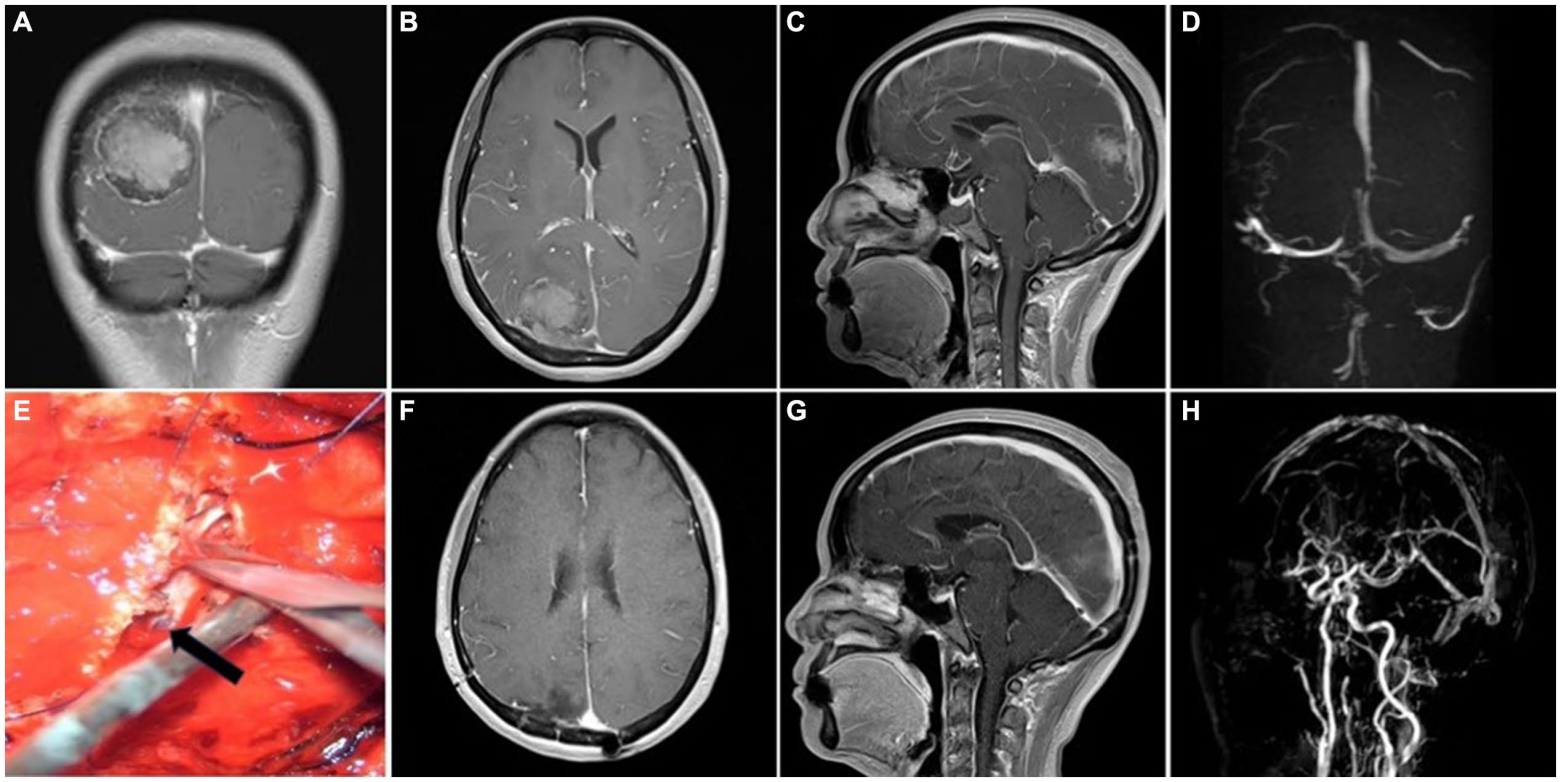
Representative case 2, type II (partial sinus obstruction). (A–C) Preoperative enhanced MRI images. (D) Preoperative MRV images. (E) Intraoperative suture repair of the sinus wall. The arrow shows the intact inner layer compressed by tumor. (F,G) Postoperative enhanced MRI images. (H) Postoperative MRV images.
